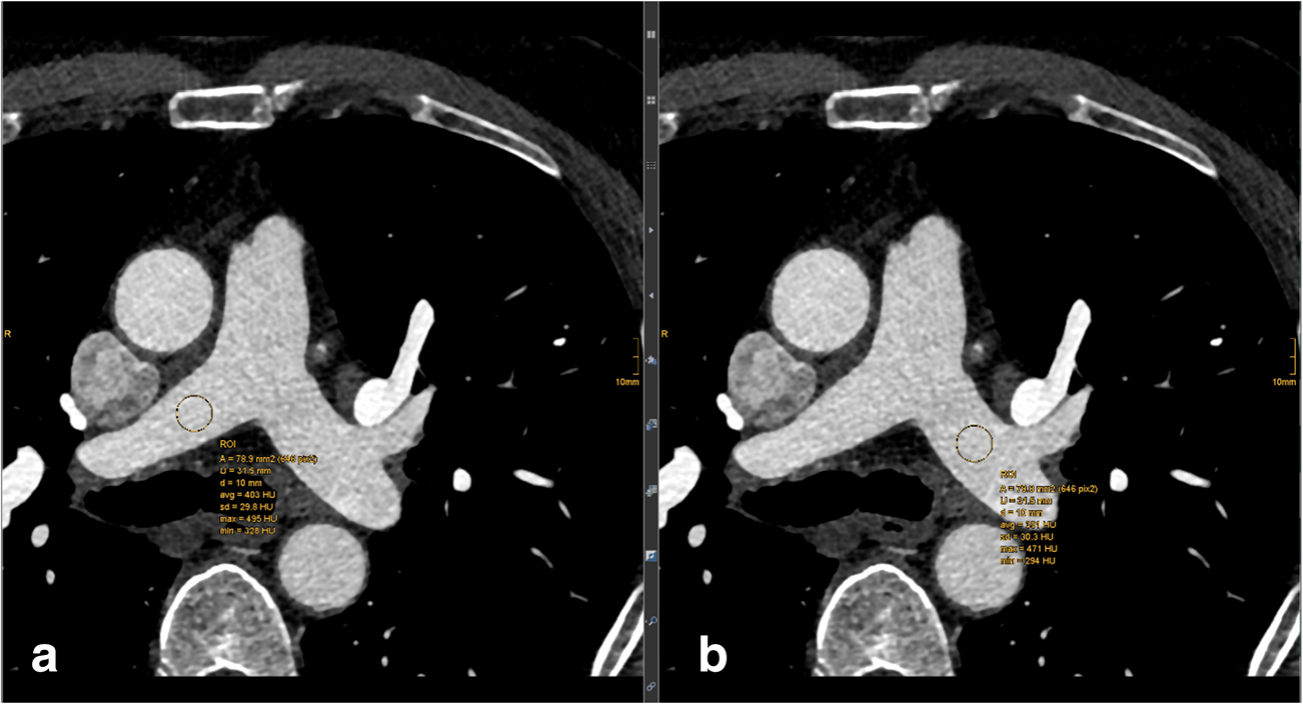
Positions of ROIs for quantitative analysis of pulmonary arteries (after completion of computed tomography angiography scan). a ROI in the right pulmonary artery; b ROI in the left pulmonary artery
